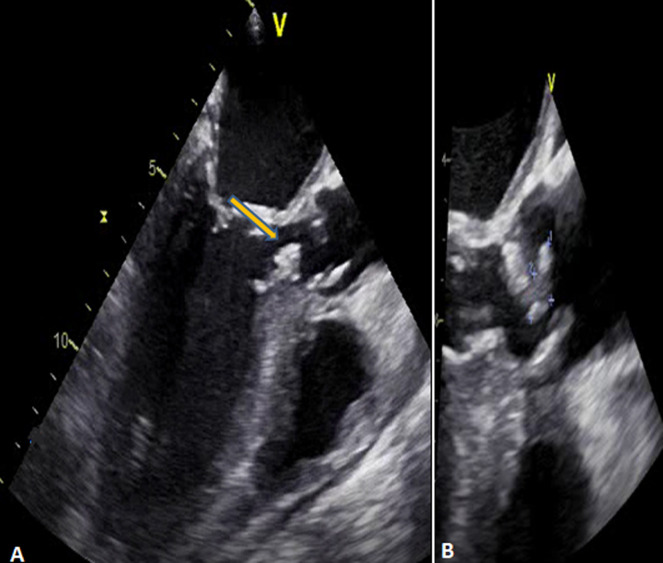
vegetations on the sub-aortic tunnel and aortic valve; transoesophageal echocardiogram 3 chamber view 120°: a thickening and an oscillating vegetation is observed in the LVOT (yellow arrow) (A); a 10*6 mm vegetation is evidenced across the aortic valve (B)
